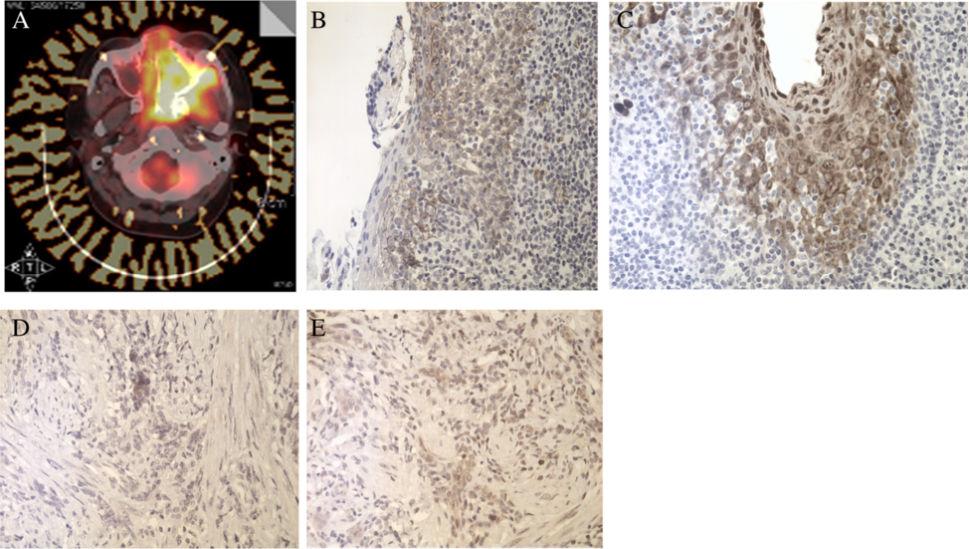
Positronemission tomography/computed tomography. (A) High 2-fluoro-2-deoxy-D-glucoseuptake in the left maxillary sinus. Expression of (B) GLUT-1, (C) HIF-1α, (D) PI3Kand (E) p-Aktwas positive.
